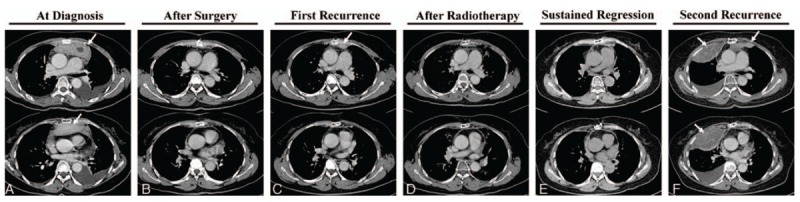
Computed tomography scans of the thorax of the patient. A: at diagnosis; B: after the first surgery; C: recurrence at 9 months after surgery; D: regression after radiotherapy; E: sustained regression for 7.5 years after surgery; F: second recurrence at 8 years after surgery. White arrows indicate tumors.
